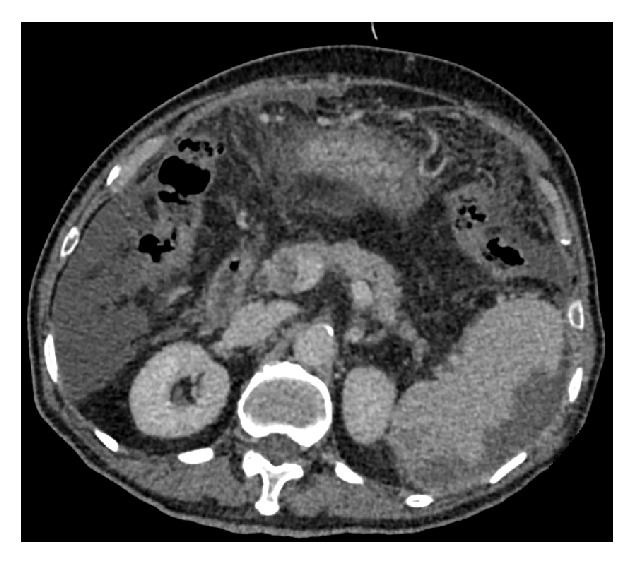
Large area splenic infarct based on CT angiography of the portal venous system.
